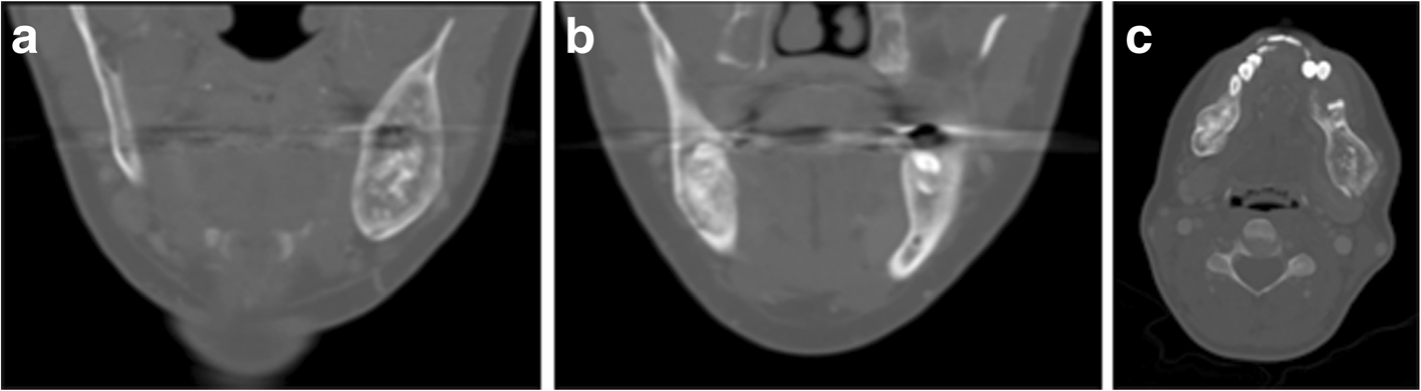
a–c 31-year-old female patient with bilateral jaw pain. CT showed bilateral mandibular brown tumours. Lesions contained stipple calcification
